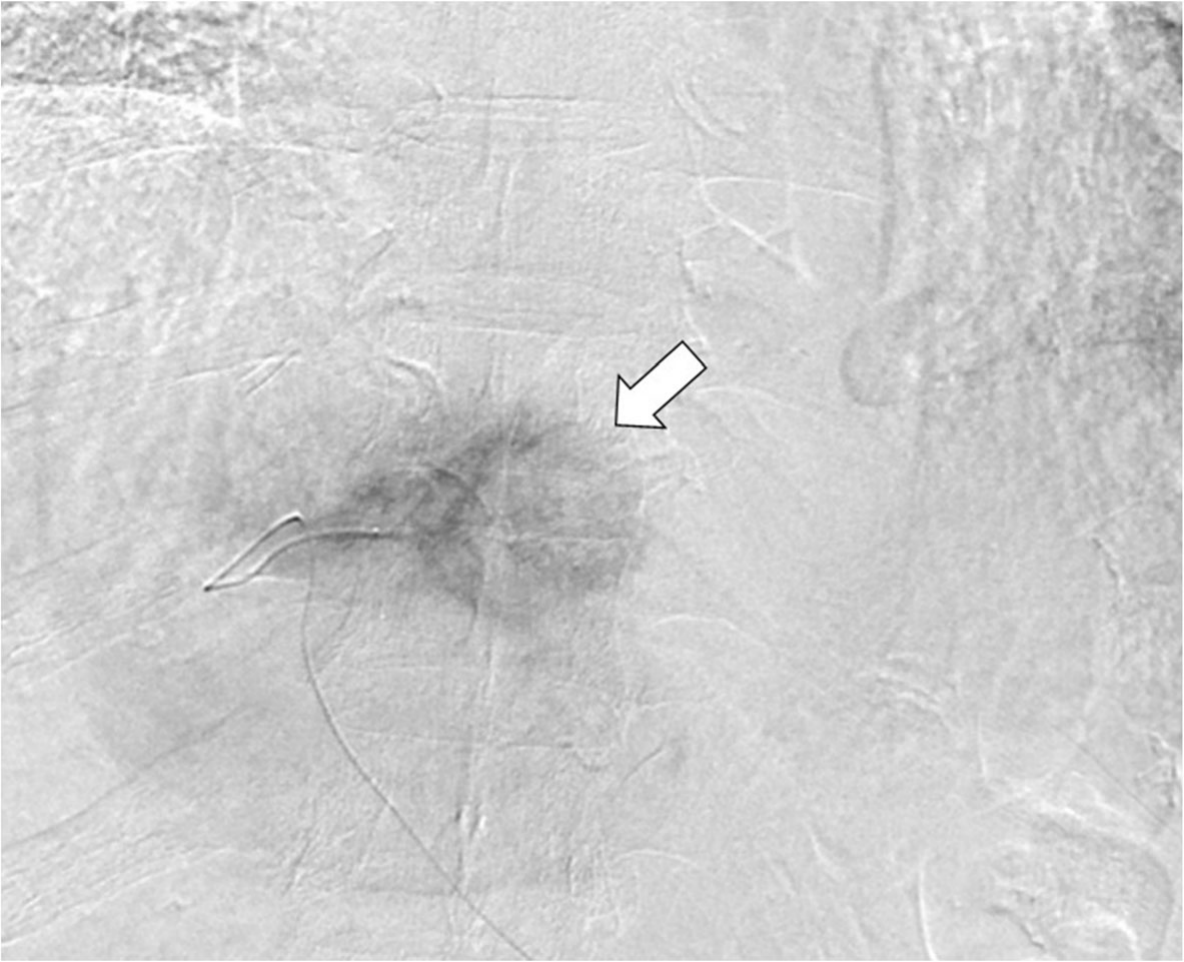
Hepatic angiogram showing a large 40-mm hepatic tumor corresponding to the lesion on plane computed tomography. The hypervascular tumor in the left lobe is depicted as a round mass of contrast opacification (*straight white arrow*) and as being supplied by the left hepatic artery
